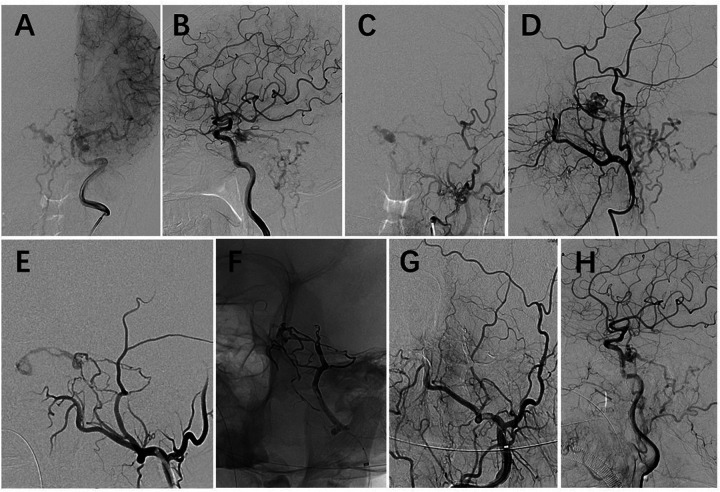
Angiography before the operation. (A) and (B): Left internal artery angiogram showing a DAVF on the left cavernous sinus supplied by the meningohypophyseal trunk and drained into the spinal perimedullary vein in continuation with intercavernous sinus and right cavernous sinus. (C) and (D): Left external artery angiogram demonstrating the same fistula also supplied by branches of the middle meningeal artery. (E): The left external artery angiogram showing branches of the middle meningeal artery feeding the fistula on the left cavernous sinus. (F): Plain radiography showing the final Onyx cast, mainly in the branches of middle meningeal artery and partially in the fistula. One coil was filled to block the distal middle meningeal artery, and Onyx-18 was injected using pressure cooker technique. (G): Injection of left external artery showing the fistula was embolized. (H): Injection of left internal artery showing the remained fistula was supplied by meningohypophyseal trunk.
